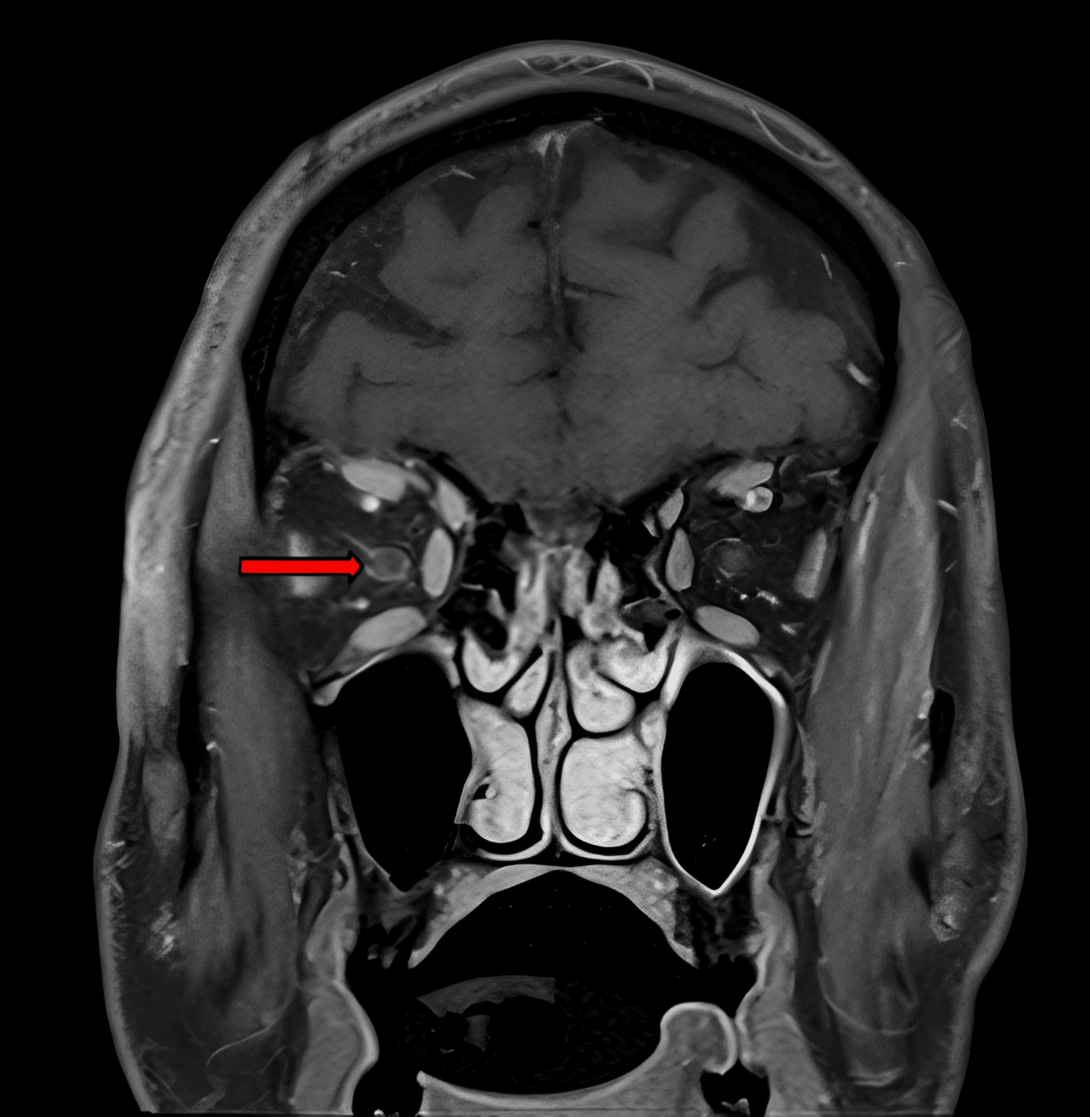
A coronal MRI image with contrast demonstrating enhancement of the perineural sheath of the optic nerve in the right eye (red arrow).
